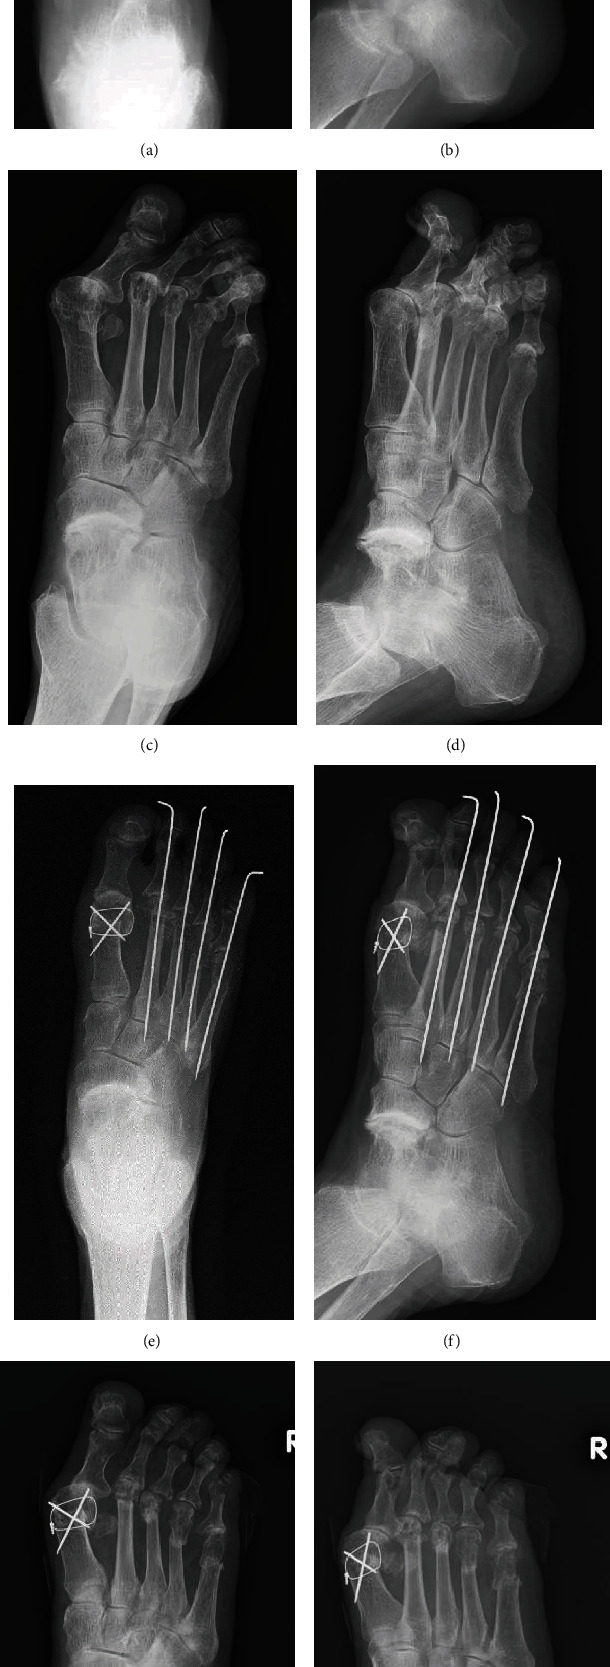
Time course of radiographs of the right foot. At 61 years old, X-ray shows that the hallux valgus angle (HVA) is 29°, the interphalangeal angle between the first and second metatarsals (M_1_M_2_A) is 9°, and the interphalangeal angle between the first and fifth metatarsals (M_1_M_5_A) is 30° (a; anteroposterior view) (b; oblique view). Just before surgery at 76 years old, HVA is 50°, M_1_M_2_A is 15°, and M_1_M_5_A is 35°, respectively (c; anteroposterior view) (d; oblique view). Mitchell's osteotomy for hallux and shortening oblique osteotomies for lesser toes were performed. Postoperative radiographs show that HVA is 19°, M_1_M_2_A is 7°, and M_1_M_5_A is 23°, respectively (e; anteroposterior view) (f; oblique view). Recent follow-up radiographs at 6 months after the surgery show that temporal Kirschner wires for lesser toes are removed and that each osteotomy site healed (g; anteroposterior view) (h; oblique view).
